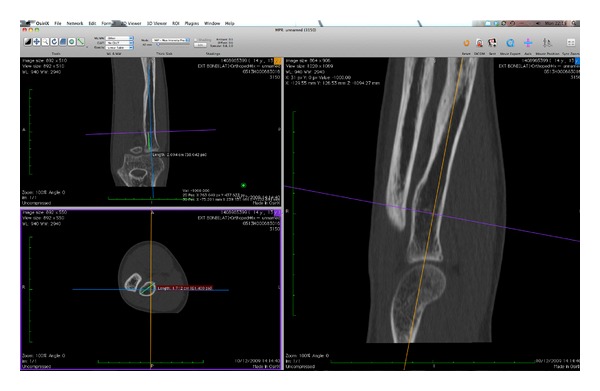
Measurements on CT scan.
